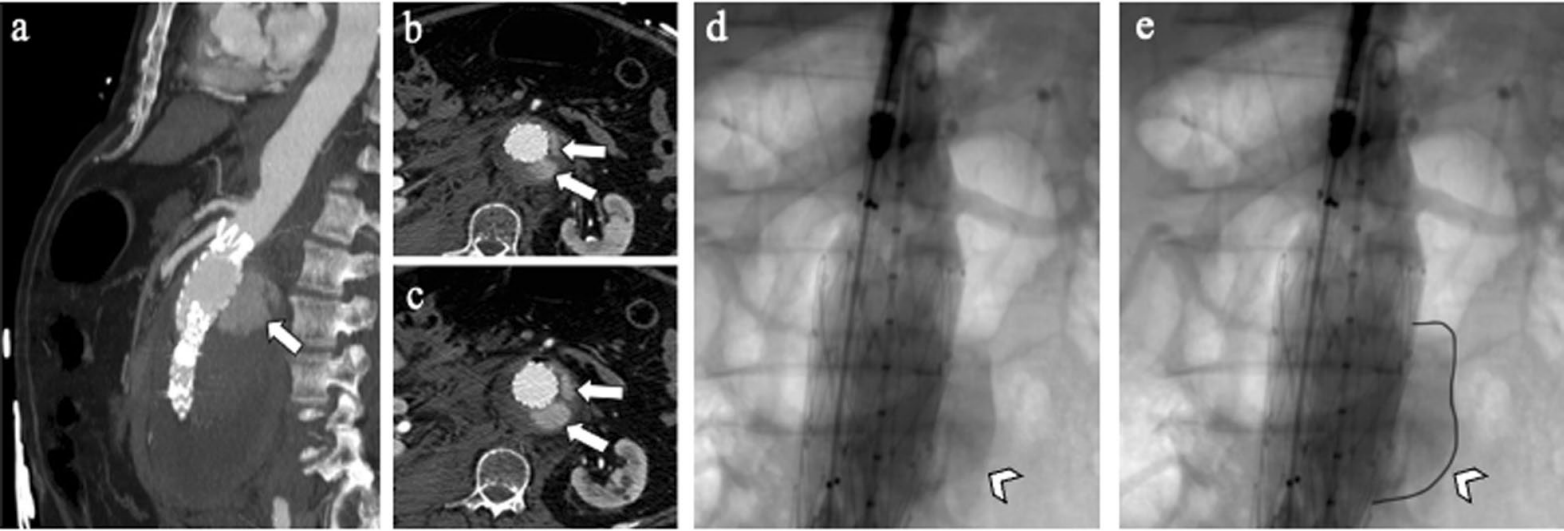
a Sagittal maximum intensity projection and (b, c) axial CT angiography images at different levels show *type Ia* endoleak in a 67-year-old man detected as contrast agent extravasation outside stent-graft within aneurysm sac (arrows), close to the proximal attachment site of the endograft; d intraoperative aortography performed by the pigtail catheter with tip positioned above the proximal endograft fixation shows a *type Ia* endoleak (arrowhead) in a 82-year-old man; e the same angiographic image shows a *type Ia* endoleak profile remarked with a black line
